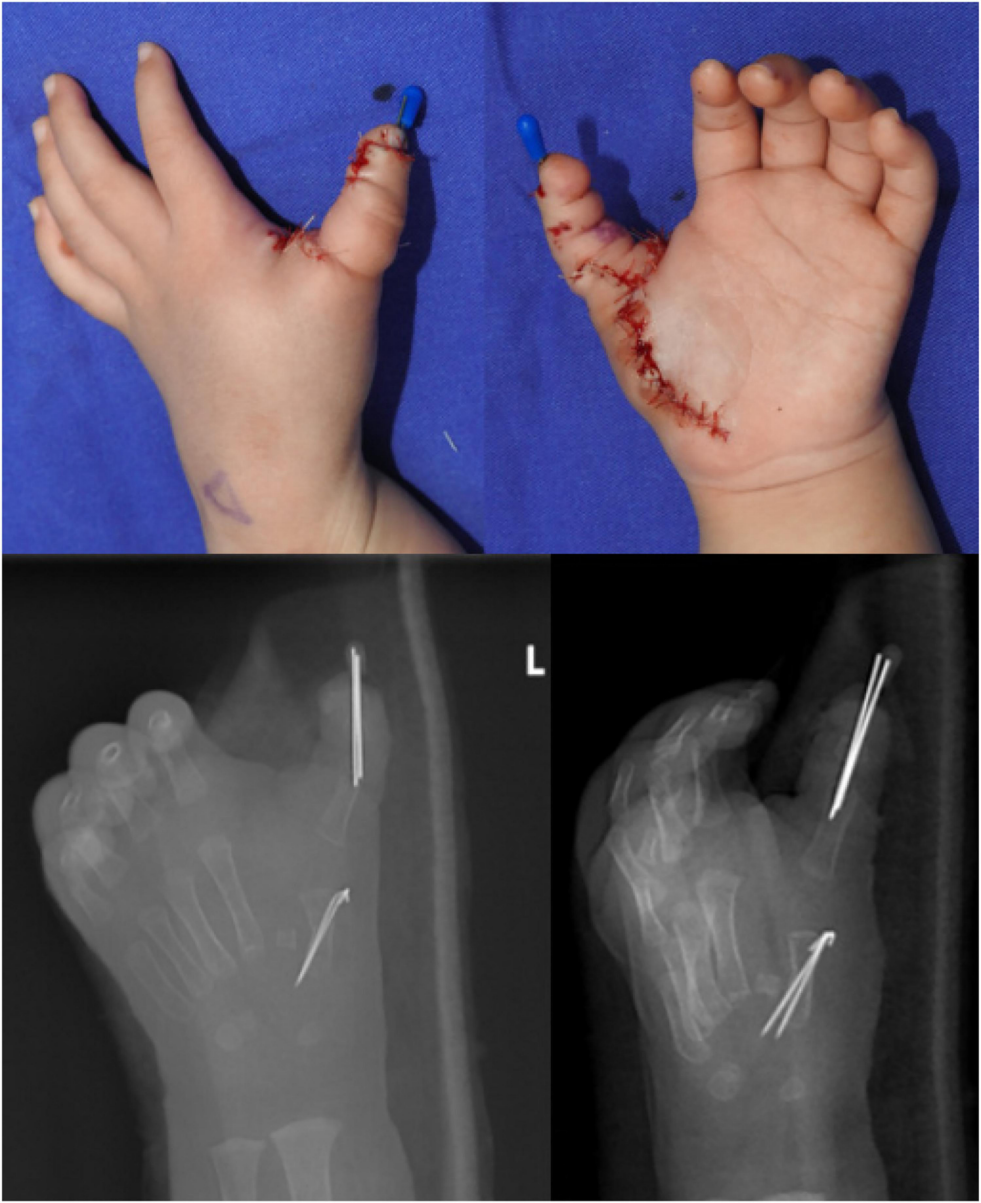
External view and orthogonal and oblique radiographs of the left hand after the operation.
